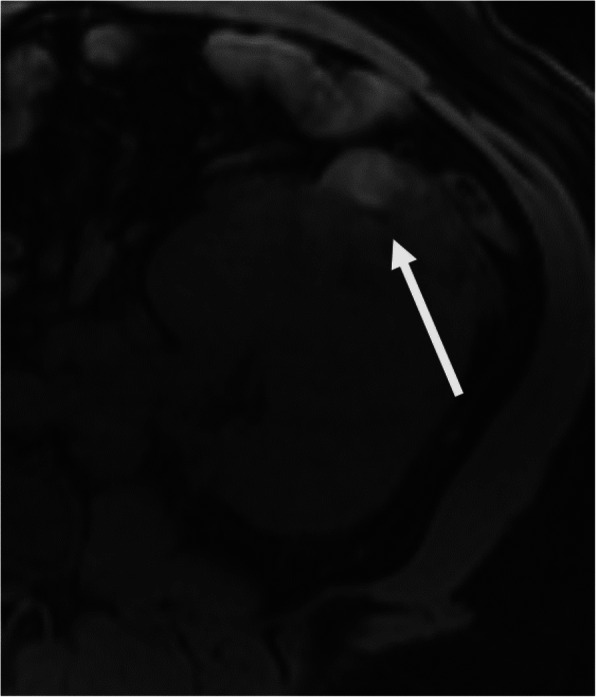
54-year-old male with fumarate hydratase-deficient renal cell carcinoma. On magnetic resonance imaging, axial non-contrast 3D fat-saturated T1-weighted sequence shows focal T1 hyperintense hemorrhage in the anterior aspect of the left renal mass (arrow)
